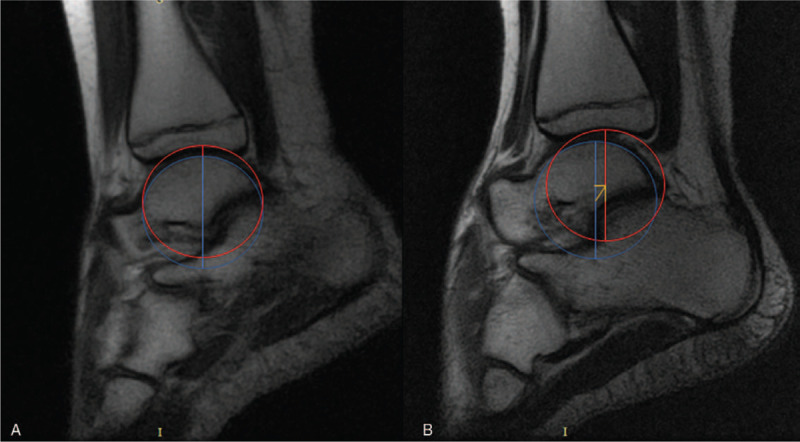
PATD assessment for anterior drawer test under MRI control. (A) Rest position and (B) after posteroanterior stress (the orange lines show the anterior, inferior and diagonal translation of the talus).
